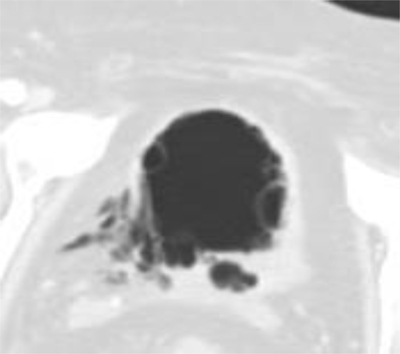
Same Axial unenhanced CT-scan image as above in Lung Window shows fibrotic tissue at the disrupton site. There are multiple air-filled submucosal blebs of the bladderwall suggesting an emphysematous cystitis.
